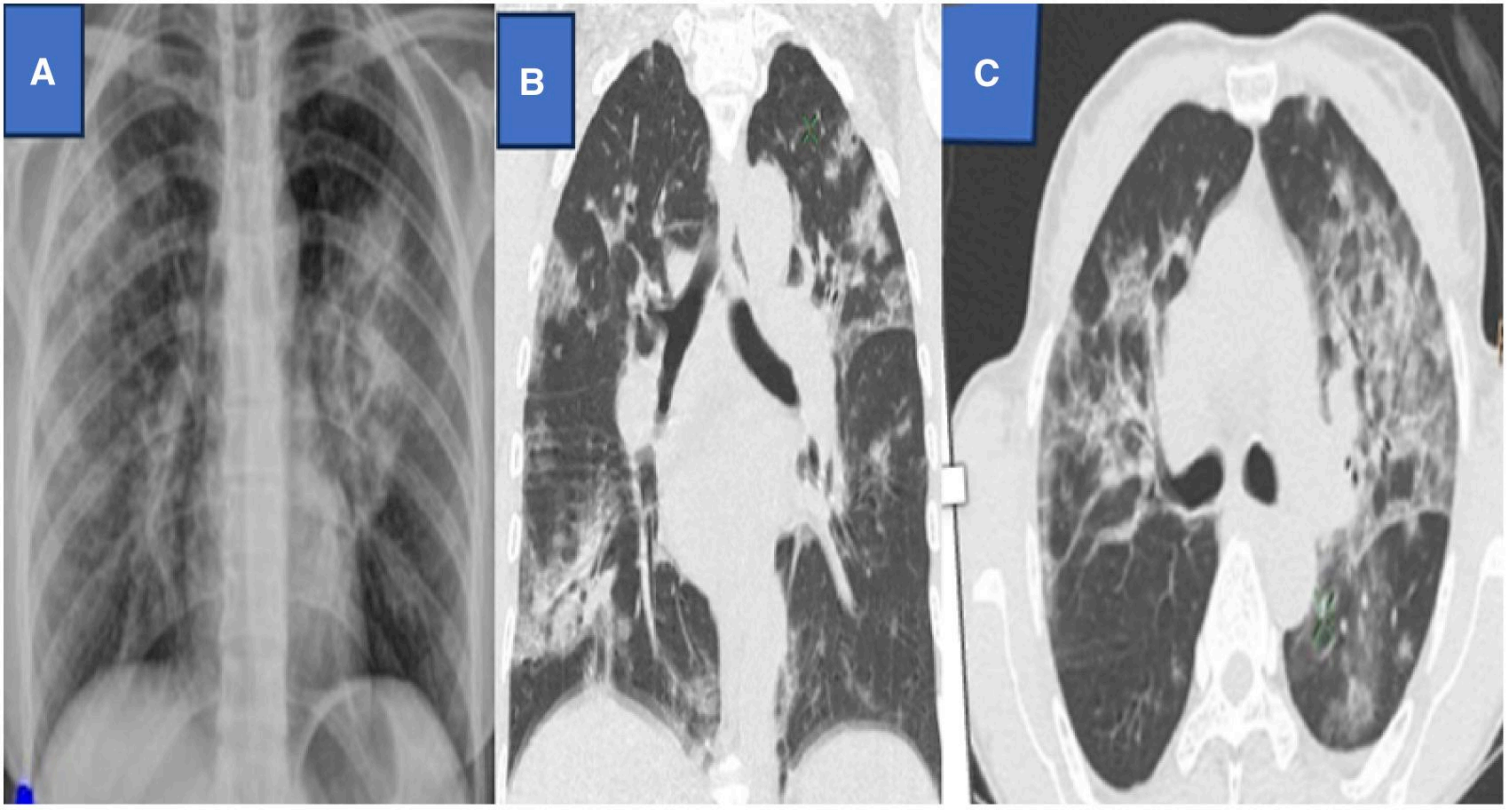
Chest X-ray (A) showed bilateral multifocal areas of airspace opacities. HRCT images (B and C) showing areas of bilateral multifocal ground-glass opacities and consolidation.
